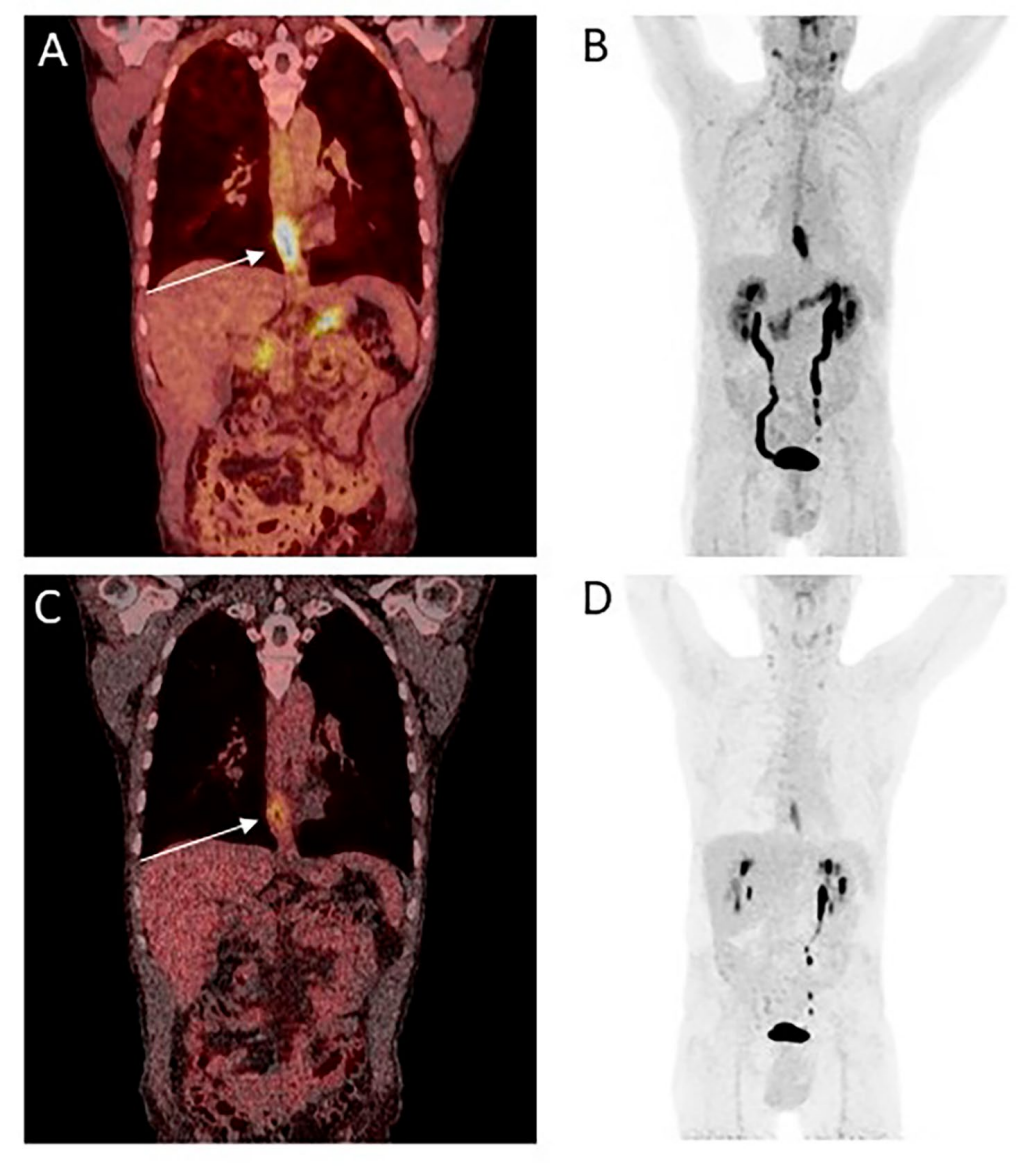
Patient with newly diagnosed distal esophageal cancer 49-year-old patient with newly diagnosed distal esophageal cancer (adenocarcinoma, G2). The primary tumor showed strong tracer uptake in the FAPI-46/dual-tracer PET/CT (A = coronal fusion, B = Maximum Intensity Projection (MIP)), whereas it shows smaller tracer uptake in FDG PET/CT (C = coronal fusion, same layer as A, D = MIP). White arrow indicating tumor. There was no sight of nodal or distant metastasis
